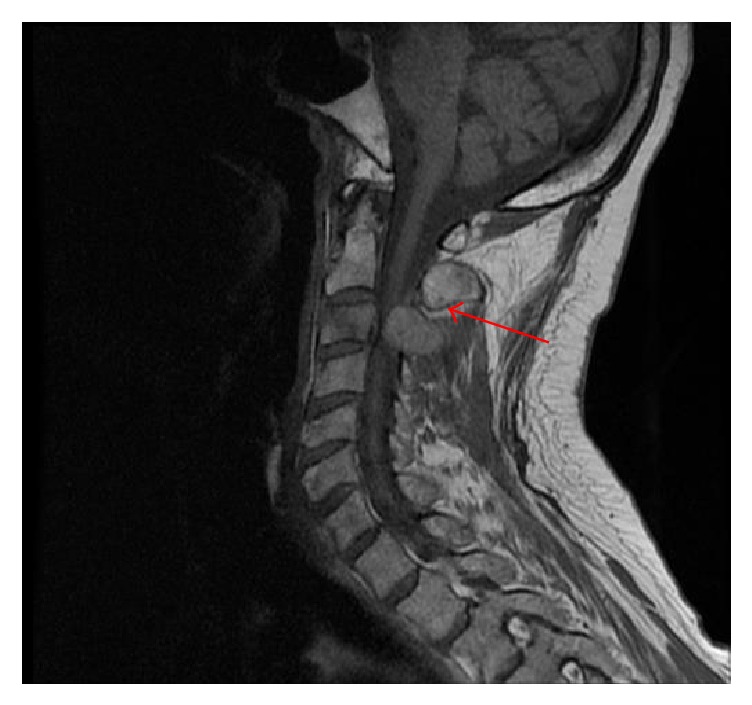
MRI of cervical spine: destructive enhancing mass at level of C2-C3 with evidence of spinal cord compression.
